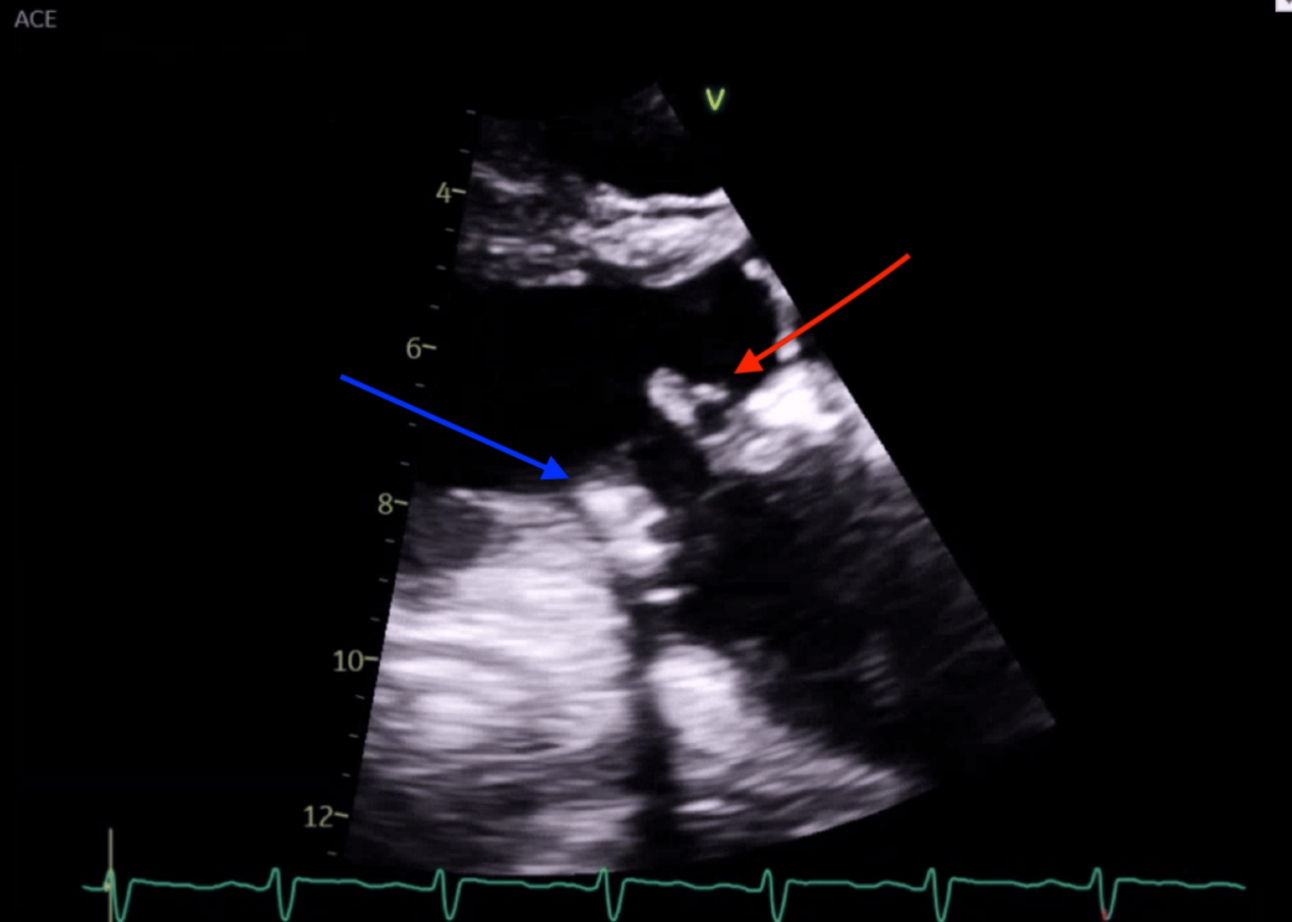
Transthoracic echocardiogram showing two highly mobile masses on hospital day 11. The mass on the anterior leaflet of the mitral valve (red arrow) with dimensions of 1.3 × 0.5 cm, concerning for infective endocarditis vs. thrombosis.
